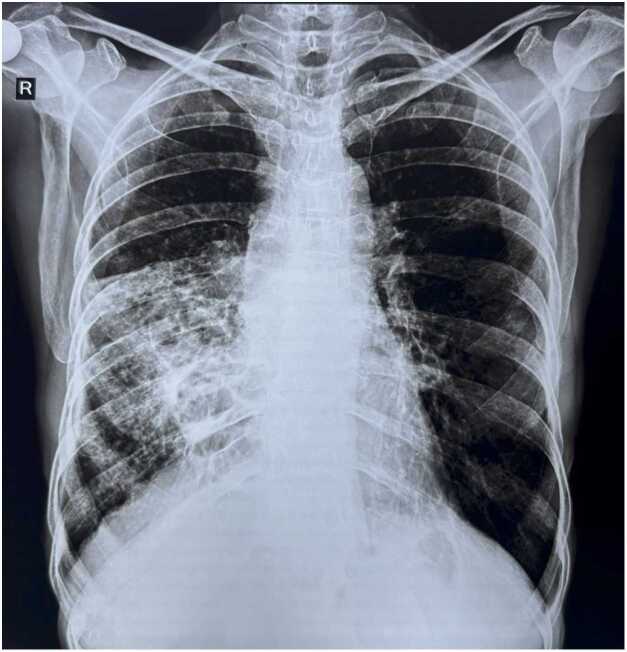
Chest X-ray posteroanterior (PA) view suggestive of right middle and lower zone heterogenous opacity.
